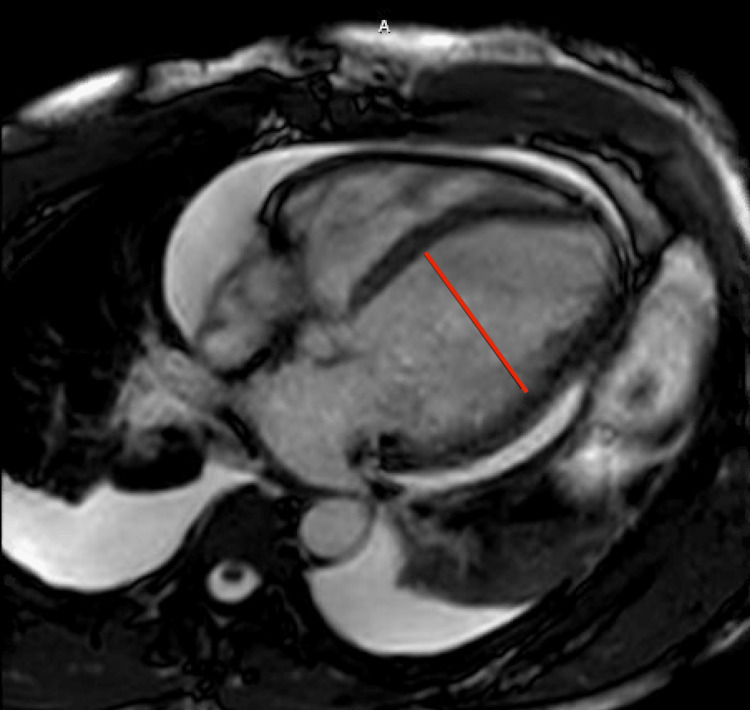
A cardiac magnetic resonance imaging four chamber view showing a dilated left ventricle with an end-diastolic diameter of 6.8 cm as seen with the red line across the short axis of the heart.
